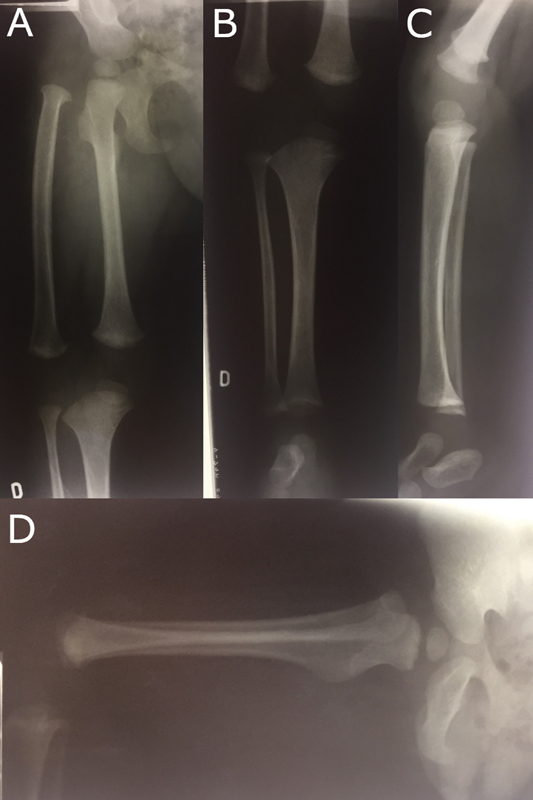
Radiograph at 1 year of age. (
